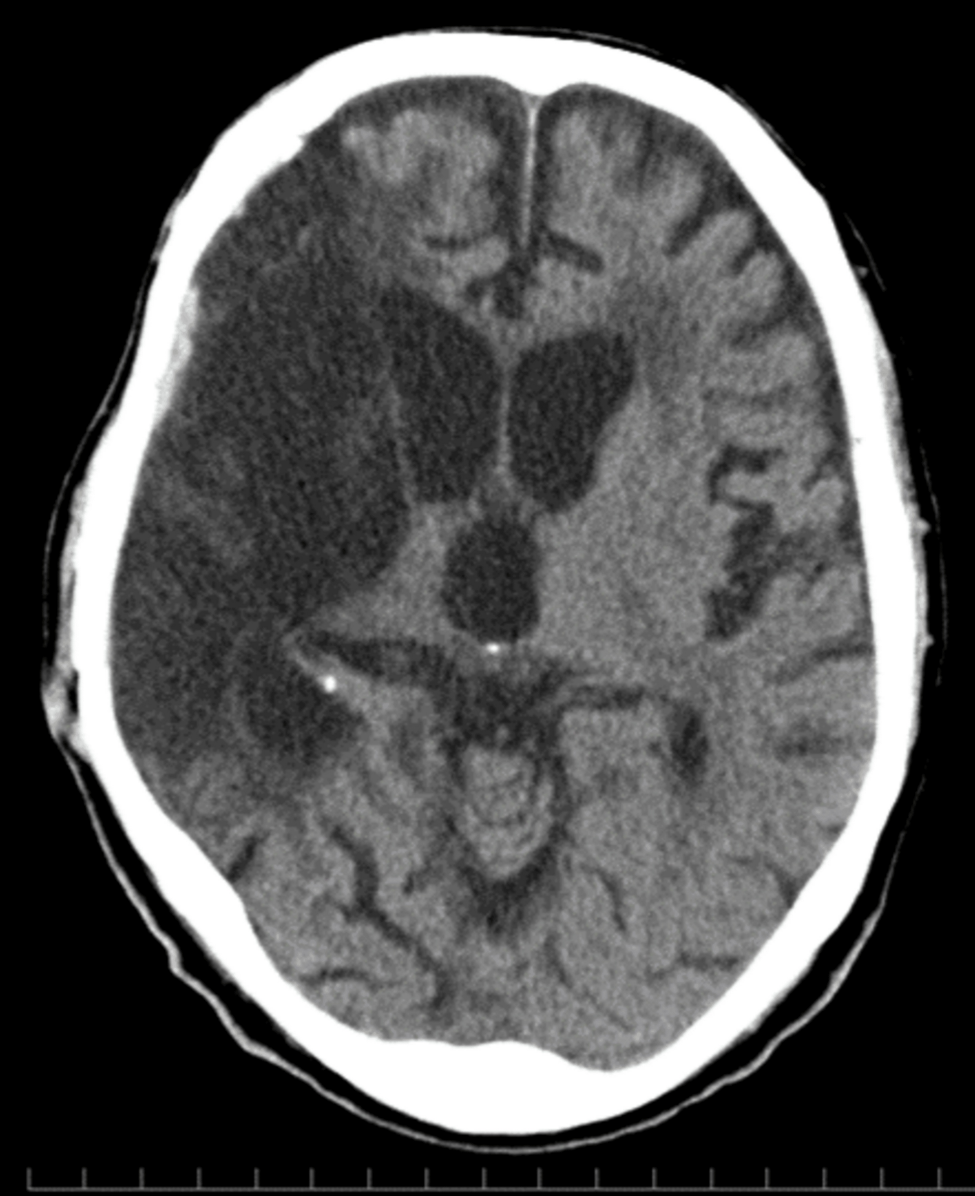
CT after LP shunt CT of the post-LP shunt showing the resolution of the subcutaneous CSF collection. CT, Computed tomography; LP, lumboperitoneal
